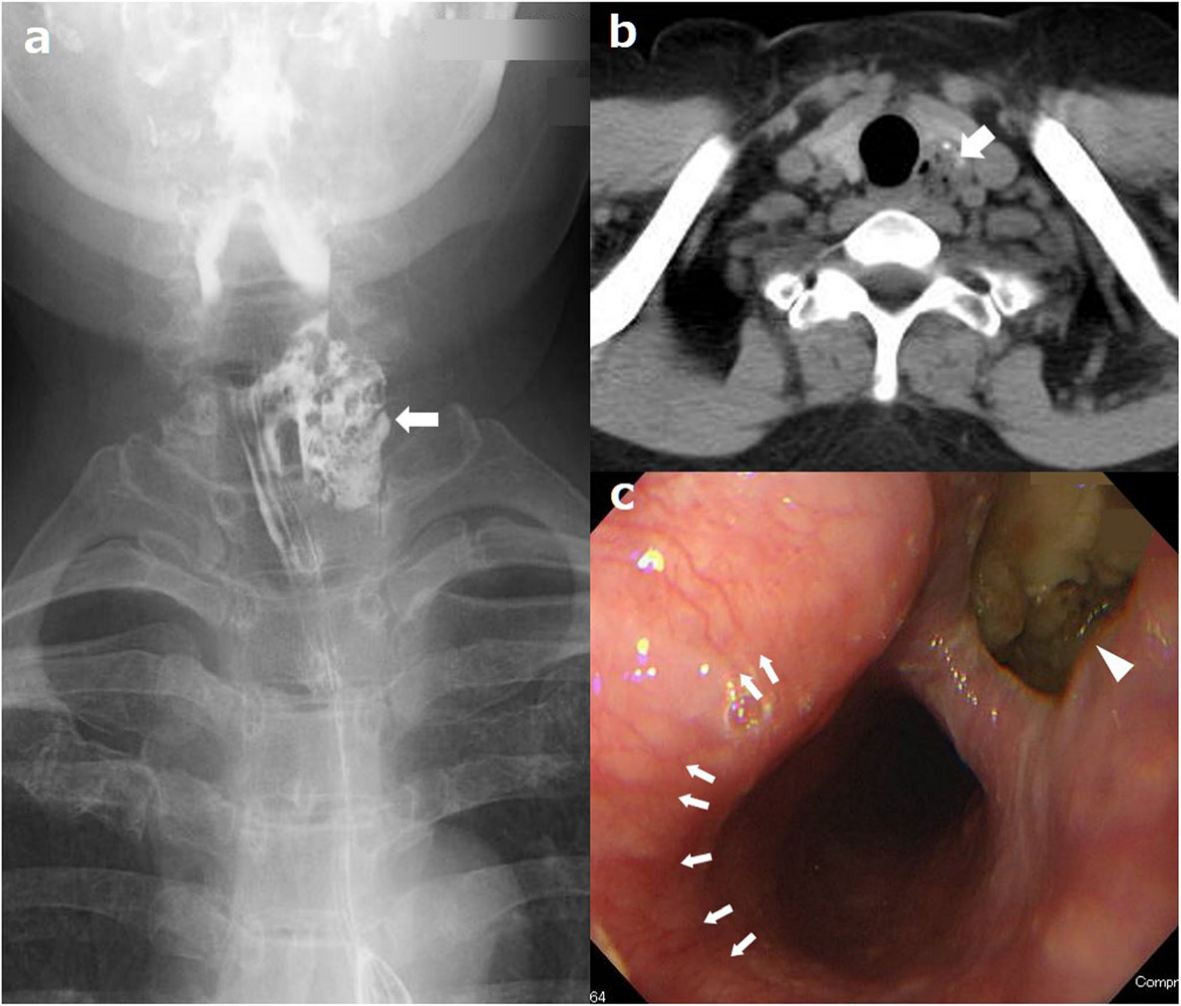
Preoperative findings. a Contrast esophagography showing a diverticulum 3 cm in diameter on the left side of the cervix (arrow) but not the cricopharyngeal bar. b Cervical computed tomography showing an air-filled diverticulum (1.6 × 1.8 × 3.2 cm) arising from behind the left lobe of the thyroid (arrow). c Upper endoscopy showing a diverticulum filled with food residue (arrowhead) just below the palisade vessels corresponding to the level of the UES (arrows)
